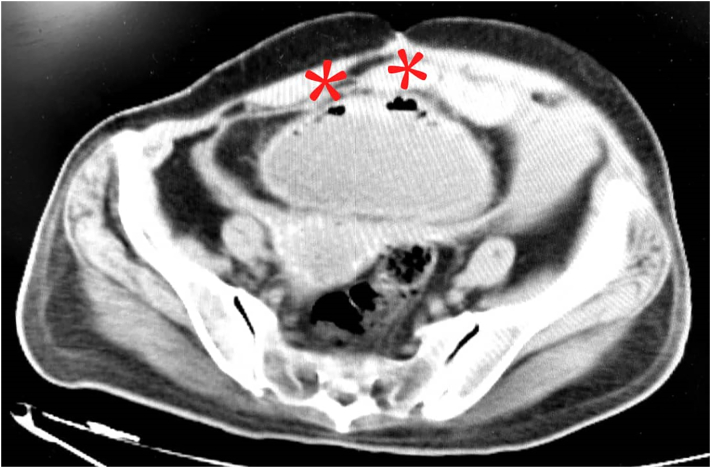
Red marks indicate gas in the bladder wall, a radiographic sign of EC. (For interpretation of the references to colour in this figure legend, the reader is referred to the web version of this article.)
